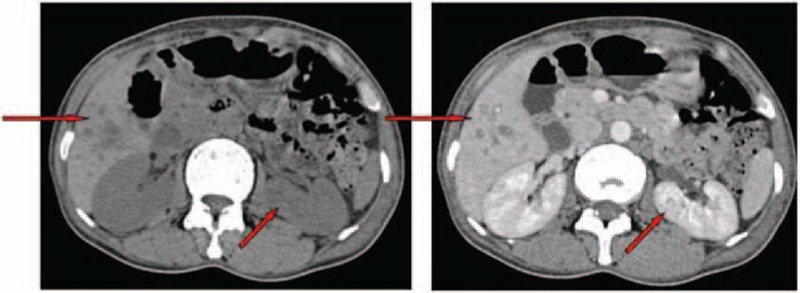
Abdominal–pelvic computed tomography scan showing multiple low density lesions in the liver and kidney.
